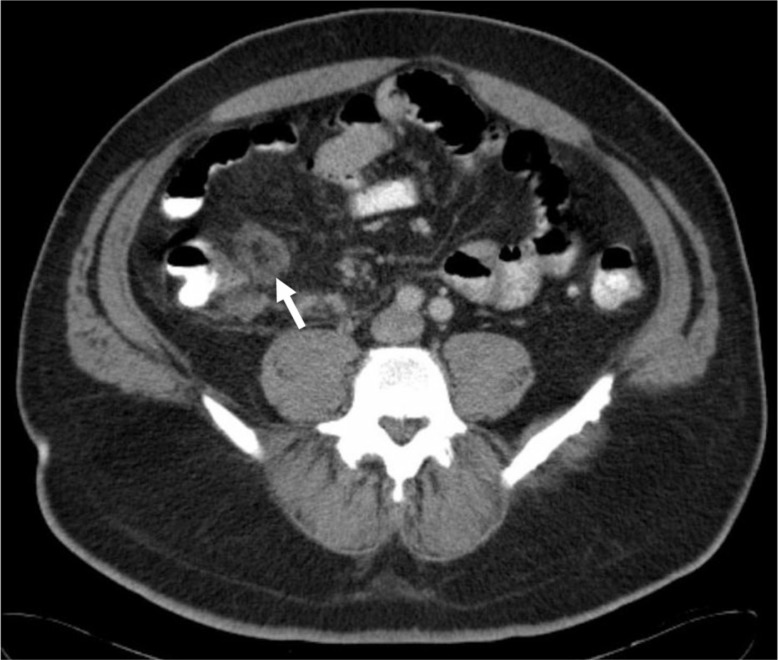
Contrast enhanced CT of the abdomen showing a focal 3.2-cm mass (white arrow) adjacent to the ileocecal valve along the mesenteric border with central fat attenuation and surrounding soft tissue attenuation suggesting epiploic appendicitis or a fatty infarct.
